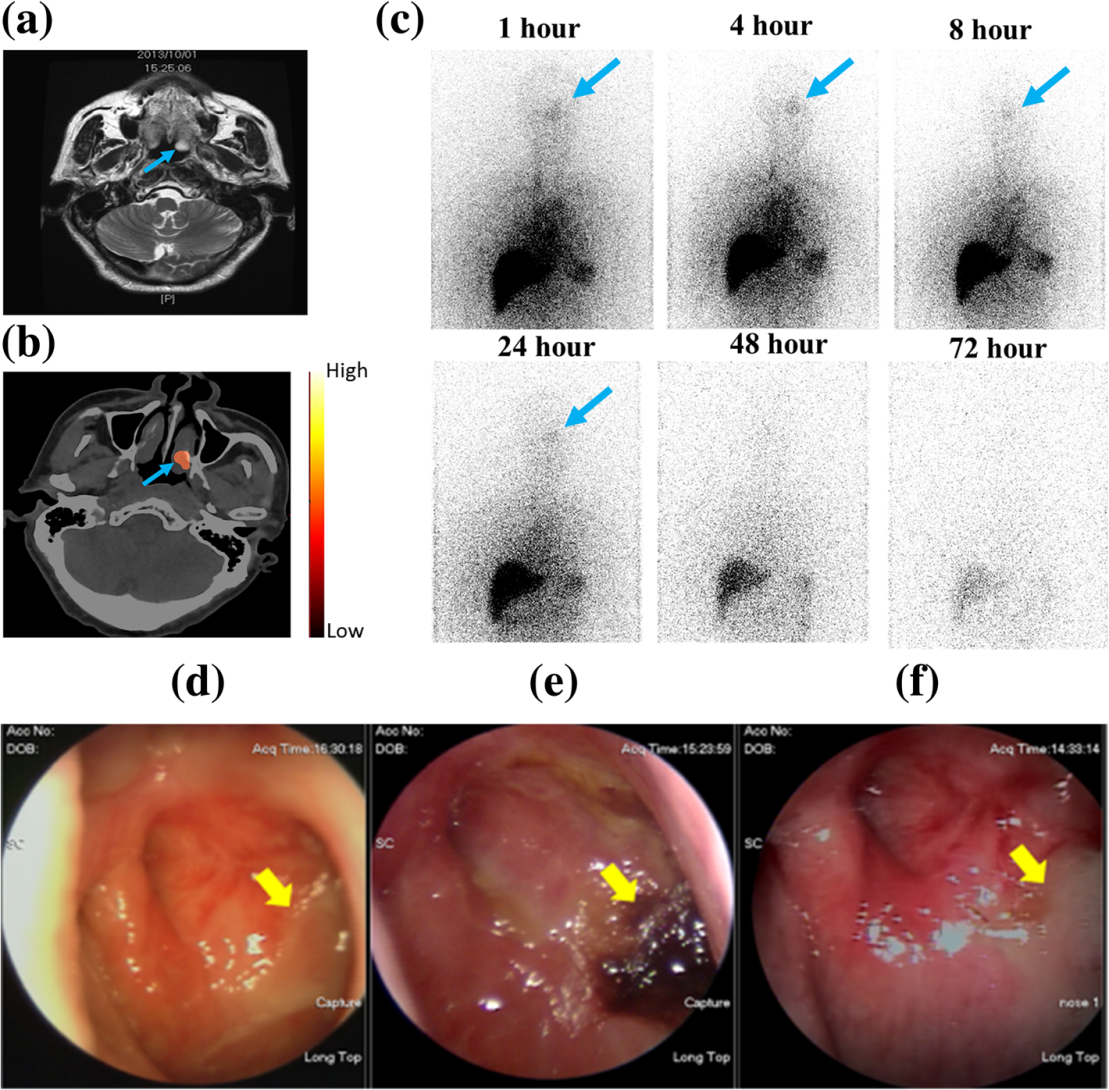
^188^Re-liposome SPECT/CT imaging showing tumor targeting and uptake in a NPC patient (Pt. 14) with pulmonary and mediastinal metastasis: a 1 month before ^188^Re-liposome injection, MRI there is a soft tissue lesion (blue arrow) in the left nasopharynx; b SPECT/CT 24 h after ^188^Re-liposome injection, there is a high uptake and efficient targeting (3.99%ID/kg) in the corresponding tumor lesion (blue arrow), as seen on the MRI; c anterior upper-body images for patient 14 at six different time points after ^188^Re-liposome injection (blue arrows indicate the lesion over the left nasopharynx); d the nasopharyncoscopic examination 1 month before ^188^Re-liposome injection shows the left nasopharyngeal mass with a crust and mucoid (yellow arrow); e 2 months after ^188^Re-liposome injection, there are engorged blood vessels and irregular surface over the left nasopharynx (yellow arrow); and f 1 year after the trial, the nasopharyngoscopic study shows fibrosis over the left nasopharynx (yellow arrow)
